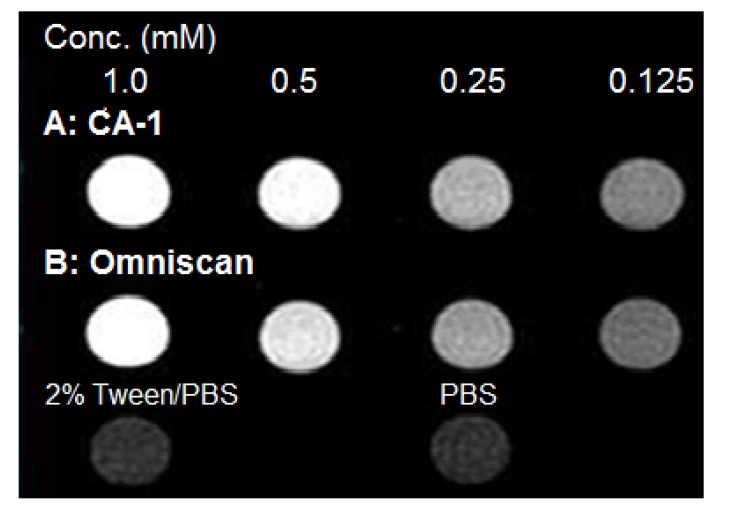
T1-weighted MRI images of CA-1 and Omniscan at 7 T and 25 °C in PBS at different Gd^3+^ concentrations.
